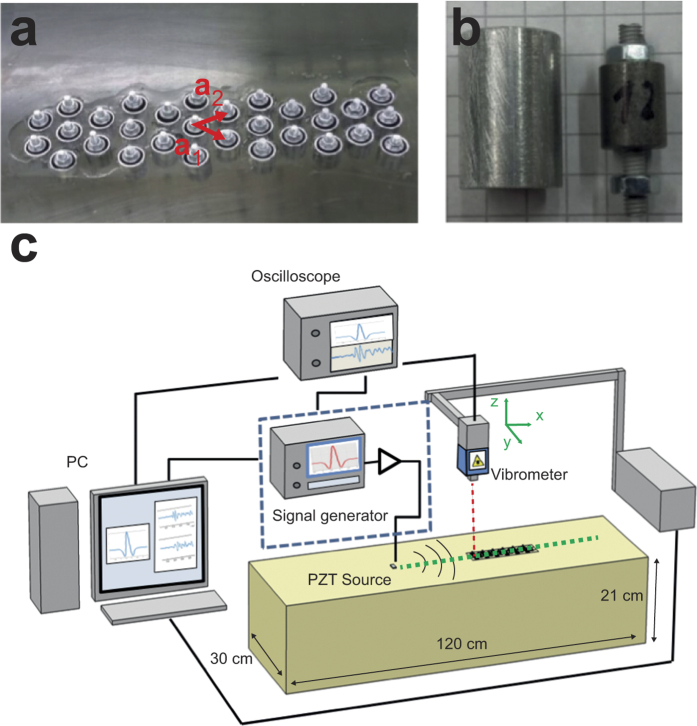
Tabletop experimental setup. (a) Resin block with embedded 30 resonators in a triangular lattice. (b) The resonator consists of rigid aluminum shell, a heavy steel mass and soft spring. (c) Experimental setup to measure the surface out-of-plane velocity wave field using a laser doppler vibrometer.
